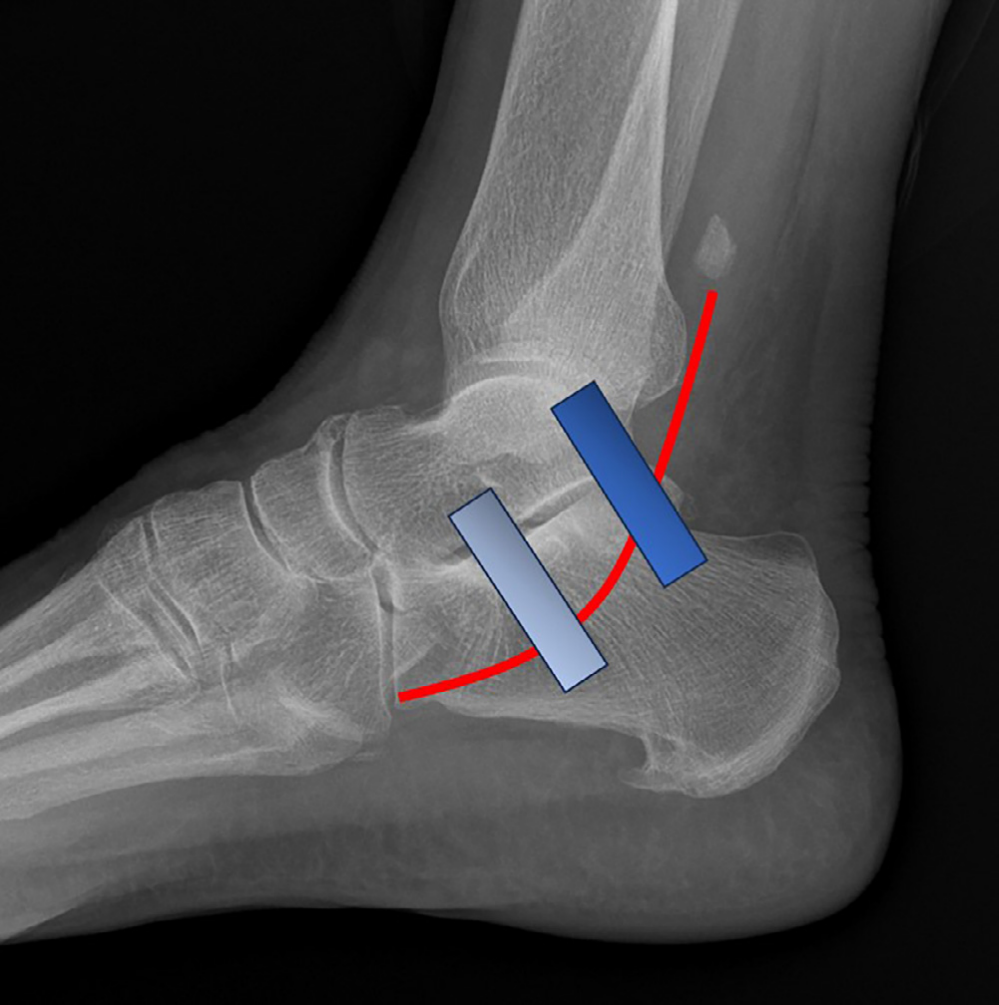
Lateral radiograph of the ankle showing the anticipated migration of the os peroneum within a retracted PLT (red curve). The location of the IPR is shown in light blue and the SPR is shown in dark blue.
